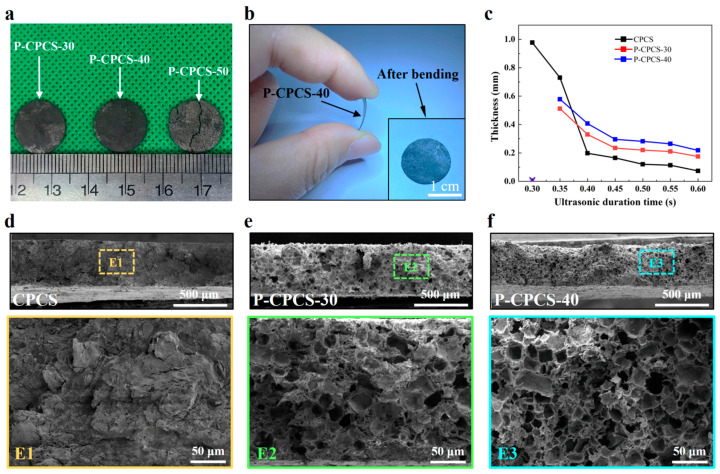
Formability and structural characteristics of P-CPCS. (a) Photographs of P-CPCS-30, P-CPCS-40, and P-CPCS-50. (b) P-CPCS-40 in bending state and after bending multiple times. (c) Variation in thickness of P-CPCS with ultrasonic duration time. Cross-section SEM images and magnified views of (d) CPCS, (e) P-CPCS-30, and (f) P-CPCS-40.
